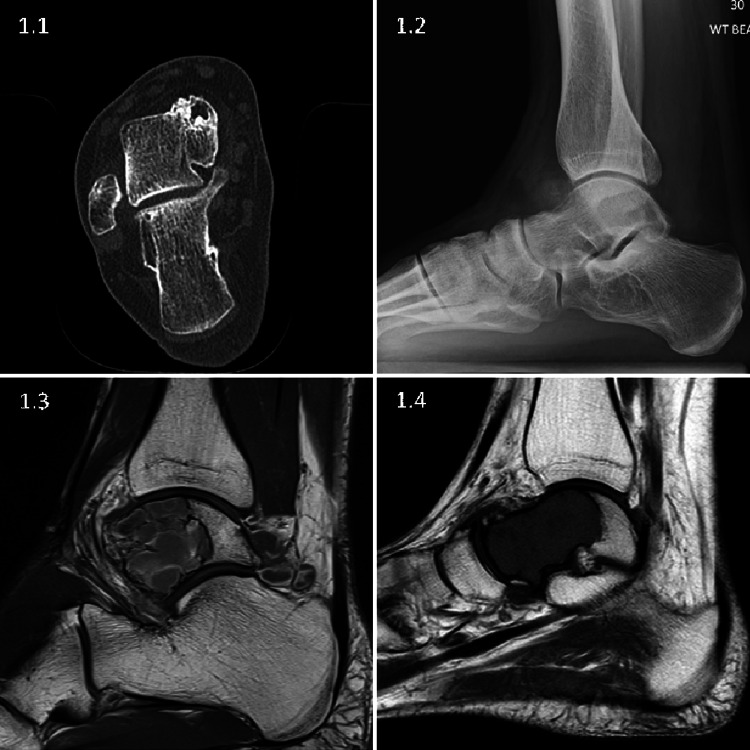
Talar bone tumours clinical images 1.1: Coronal CT scan of an osteoid osteoma demonstrating the nidus and the additional value of CT. 1.2: Lateral radiograph of an osteoblastoma, demonstrating difficulty to identify these lesions on plain radiography. 1.3: Sagittal section MRI scan of a chondroblastoma. 1.4: Sagittal section MRI scan of giant cell.
